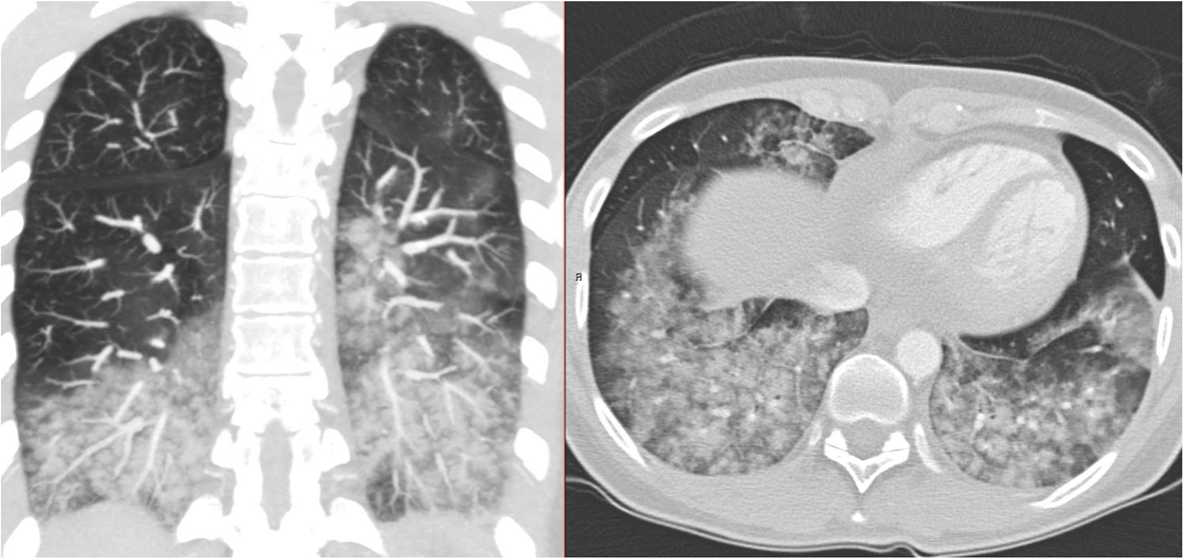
Computed tomography of the chest taken 11 h after onset of hemoptysis showing bilateral alveolar opacities
